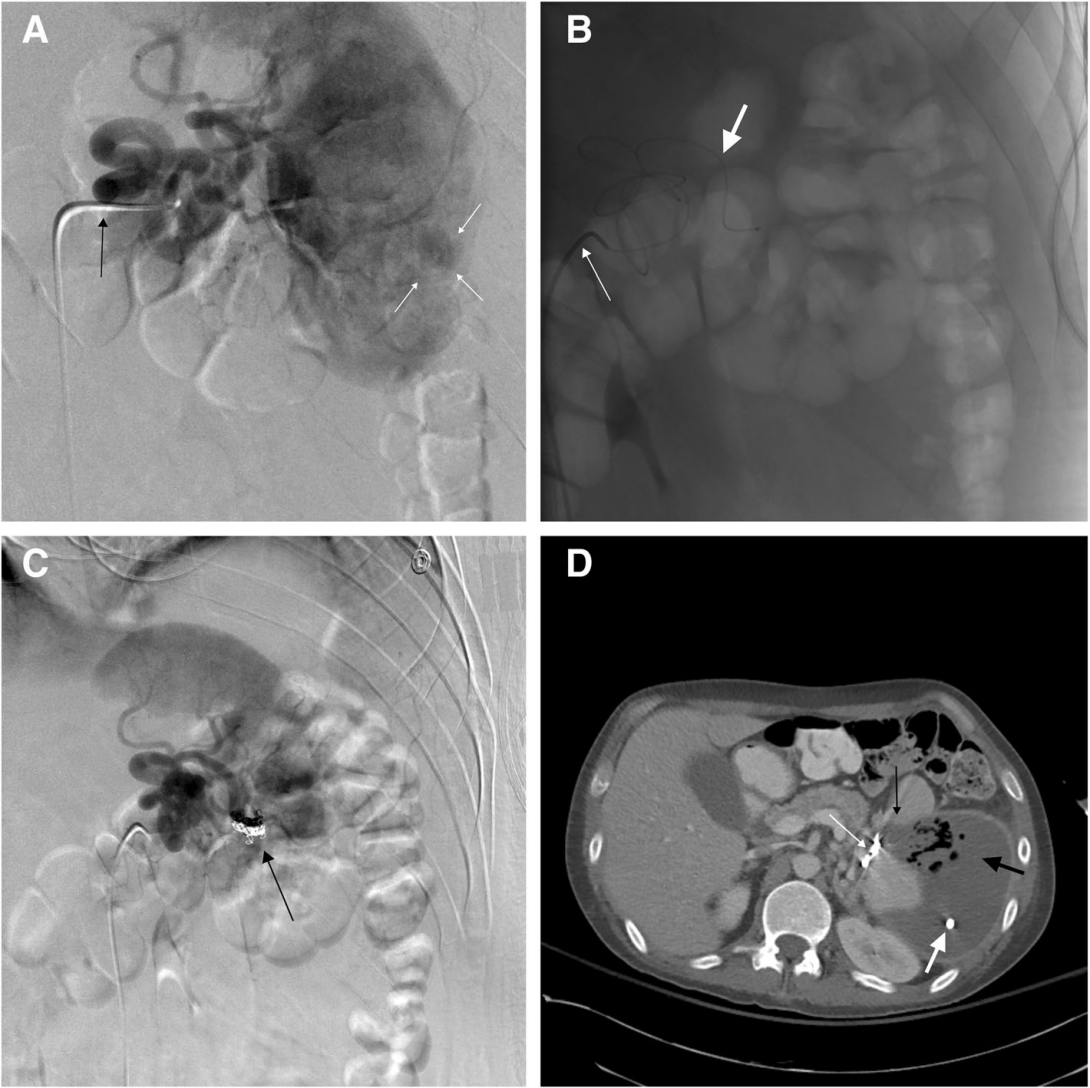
37 year old male with an AAST grade III splenic injury following high speed motor vehicle crash. a Splenic artery DSA with a catheter in the splenic artery (black arrow) demonstrating a focal pseudoaneurysm (white arrows). b A base catheter in the splenic artery (small white arrow), with a 5 F microcatheter fed through a tortuous splenic artery (large white arrow). The tortuosity and distance of this pseudoaneurysm precludes the use of traditional covered stents in this area. c Post coil embolization DSA showing a treated pseudoaneurysm. d One week after embolization, an axial contrast enhanced CT through the spleen demonstrates distal embolization coils (small white arrow), an area of focal infarction (small black arrow), and a splenic abscess (large black arrow). A partially visualized drain is present within the infected collection (large white arrow)
